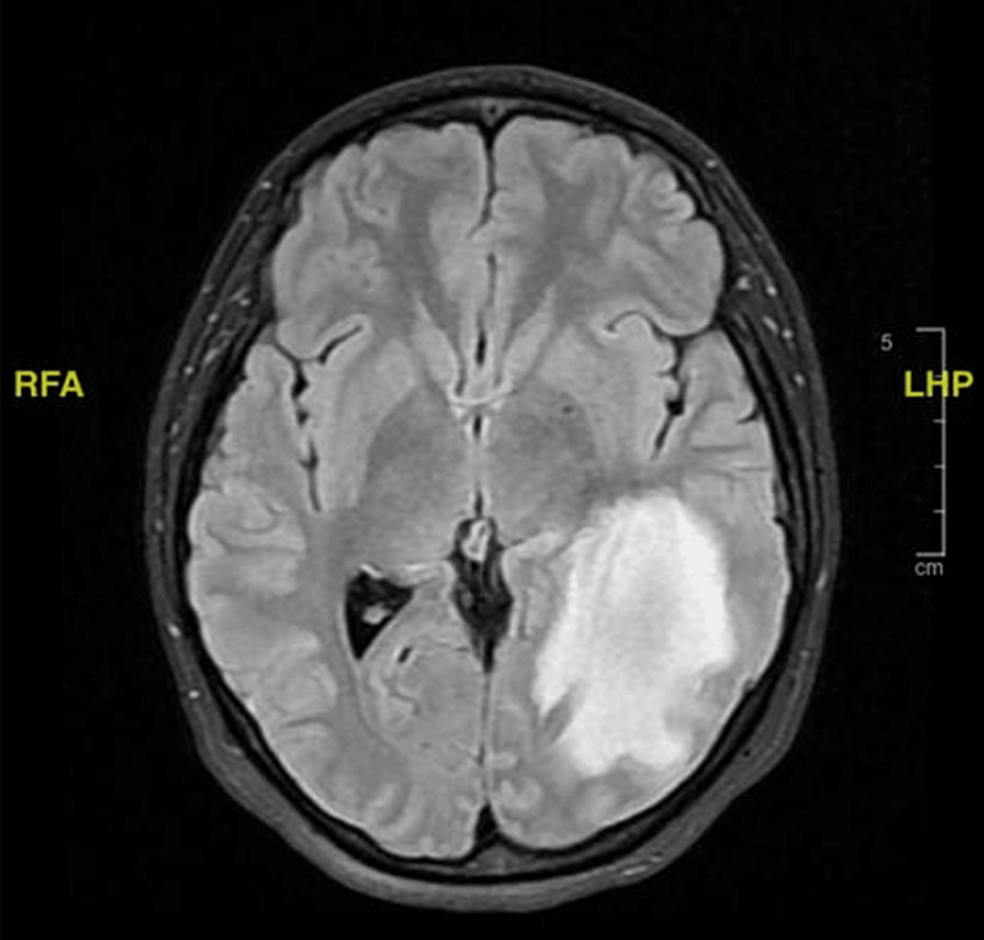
A FLAIR sequence MRI in axial view displaying a comparative regression in the size and signal intensity of the abnormal lesion seen on the left parietal lobe from previous studies. FLAIR, fluid-attenuated inversion recovery; MRI, magnetic resonance imaging; LHP, left, head, posterior; RFA, right, foot, anterior
